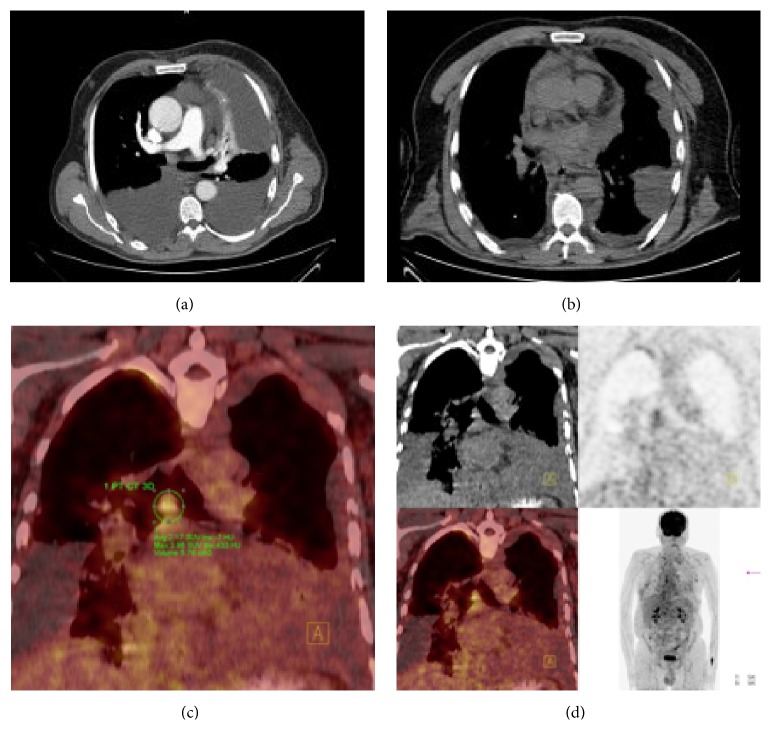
(a) A bilateral pleural effusion is noted in the CT obtained during first presentation. (b) A CT scan shows no nodular formation in the pleural surfaces following the drainage of pleural effusion. (c) A coronal PETCT image at the level of the mediastinal nodes. (d) PET-CT images are shown.
